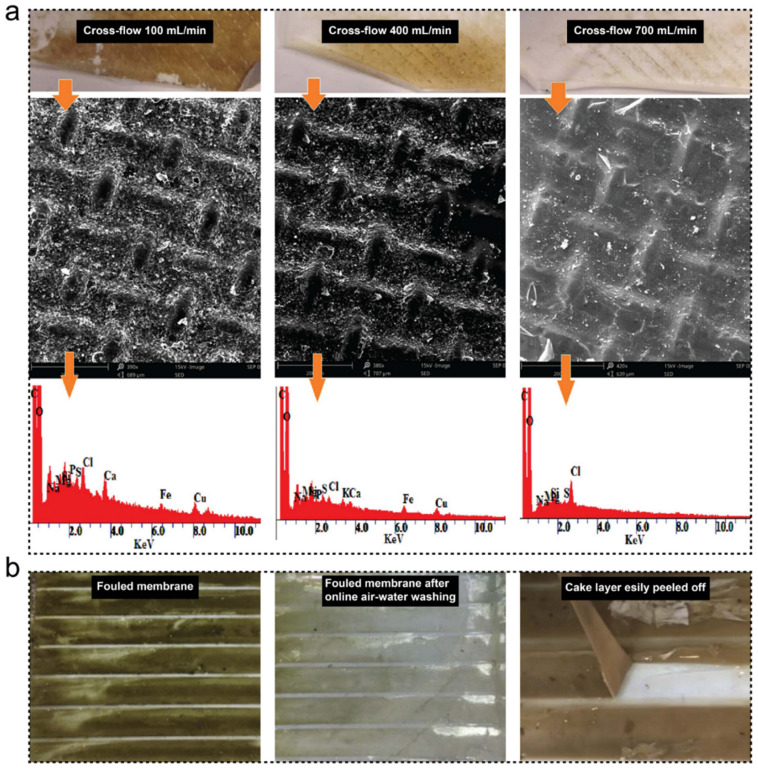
(a) Fouling behaviours of CTA-FO membranes with different cross-flow velocities, reprinted with permission from [48], and (b) photographs of the fouled membrane (left), fouled membrane after online air-water washing (middle), and visualisation of the easily peeled-off cake layer, reprinted with permission from [35].
